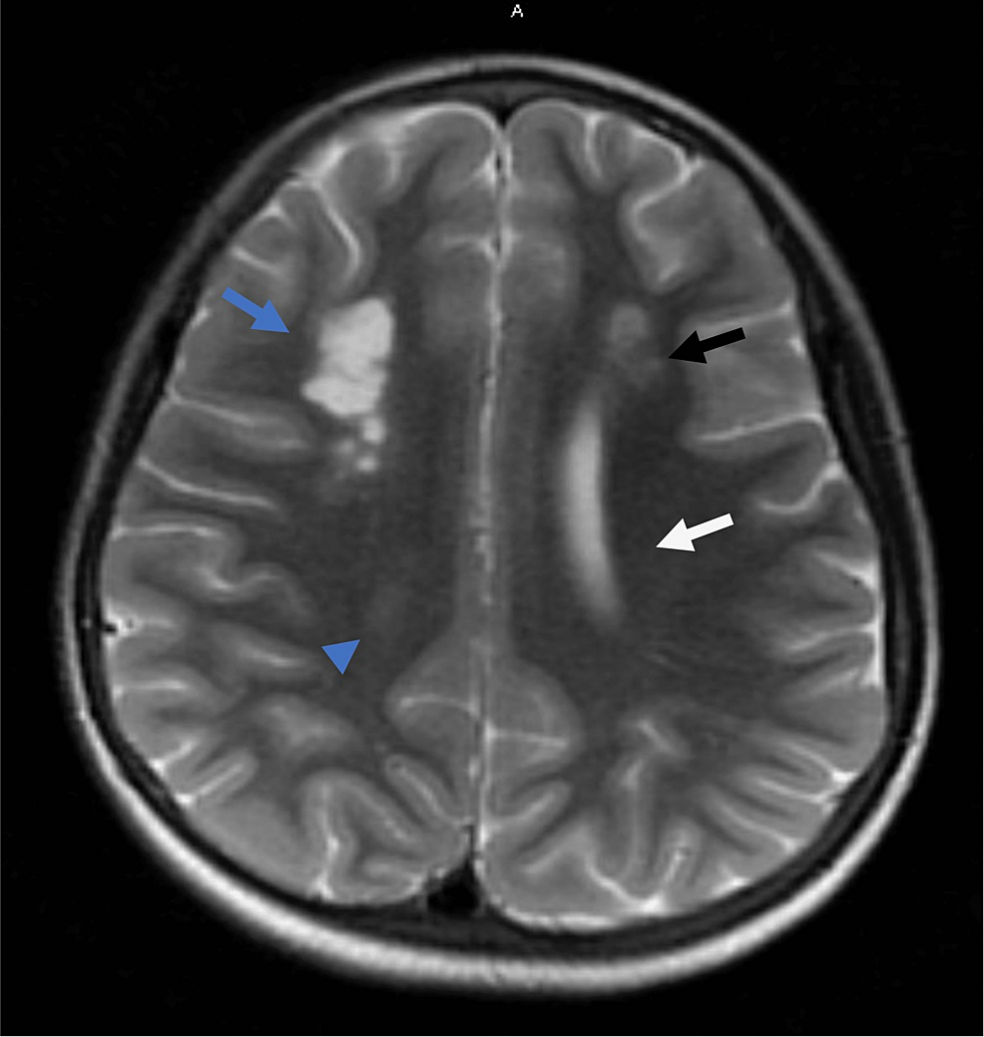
MRI of the brain with areas of restricted diffusion and T2 hyperintensity compatible with acute infarction in the left centrum semiovale (white arrow), left frontal subcortical white matter (black arrow), and, to a lesser extent, right centrum semiovale (blue arrowhead). Areas of chronic infarction can be seen in the right frontal watershed junction (blue arrow).
